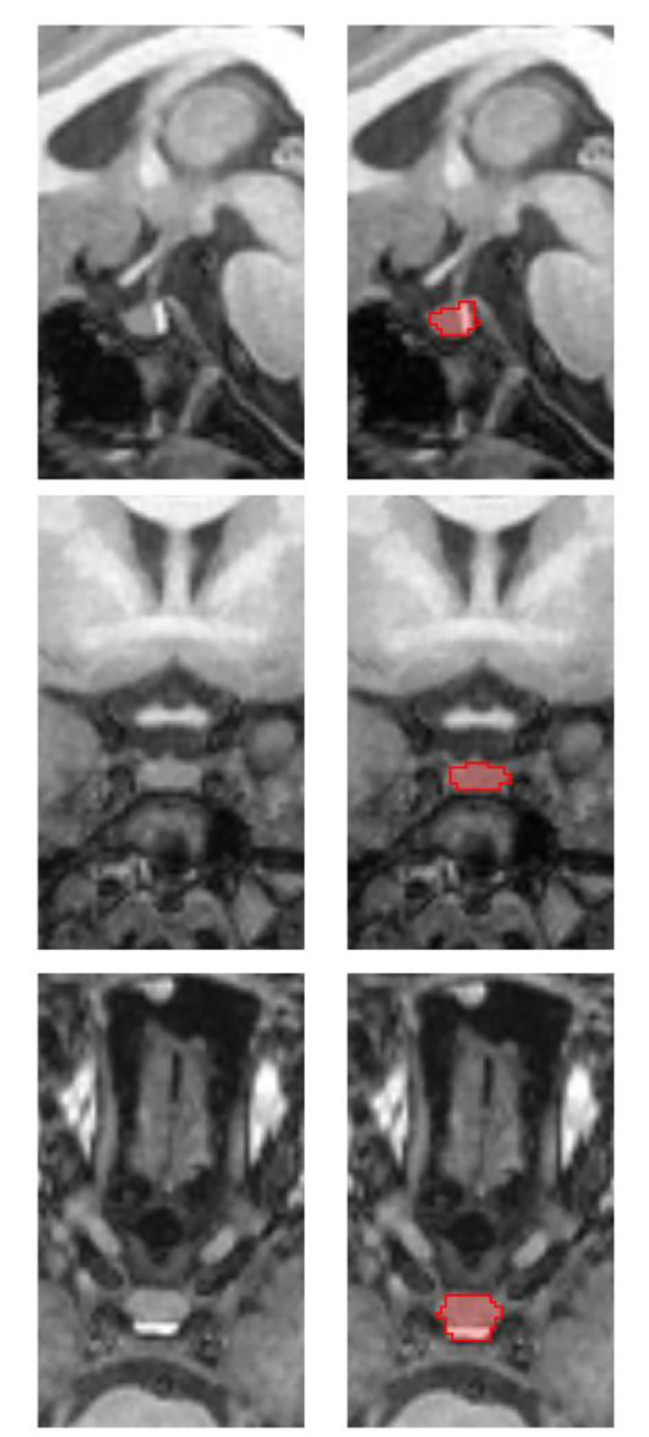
Sagittal (top), coronal (middle), and axial (bottom) view of the pituitary gland from a T1-weighted MR image. The pituitary is manually labelled in red on the right
